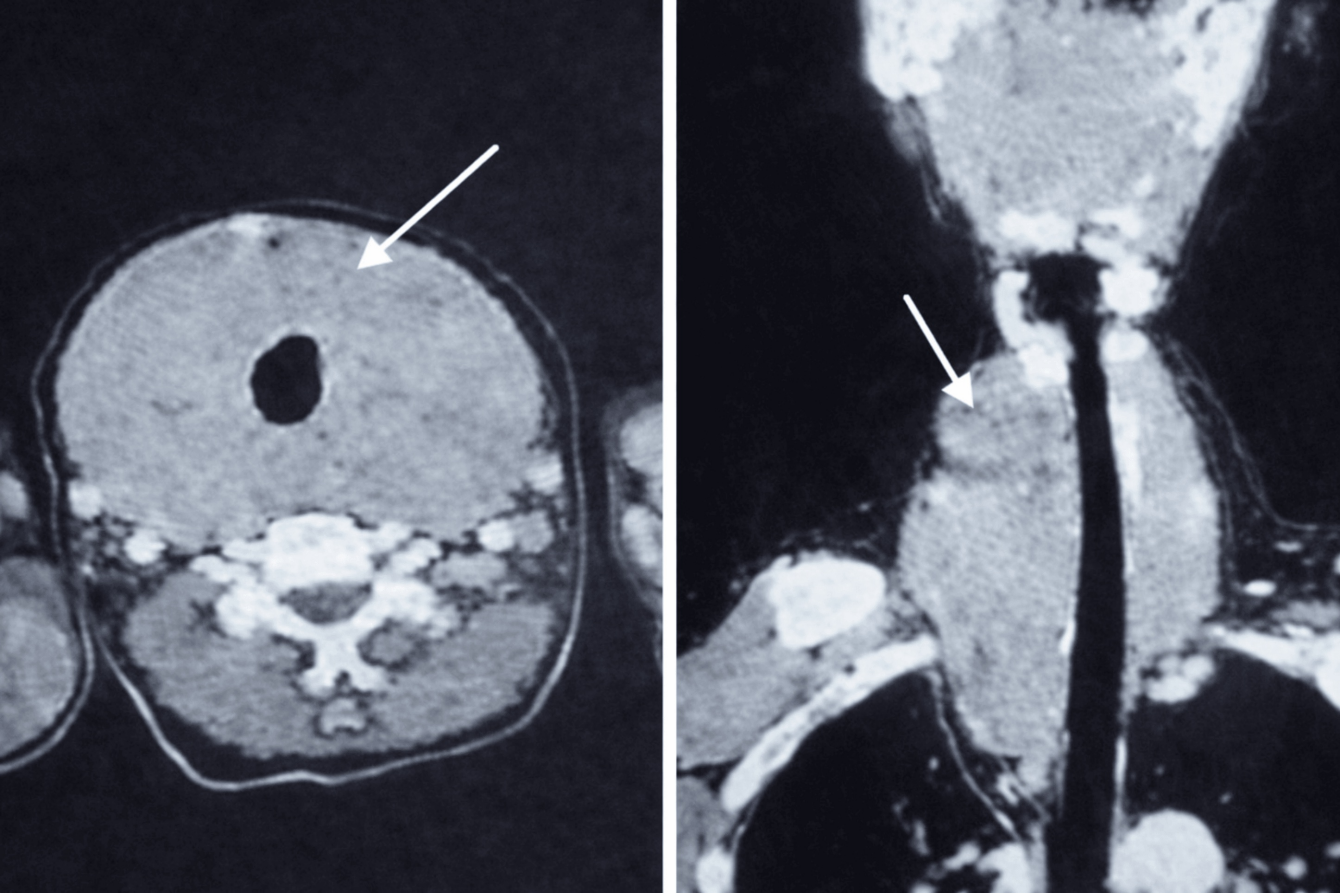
CT images showing a thyroid mass extending into the superior mediastinum and causing deviation of the trachea White arrows indicate the location of the mass.
